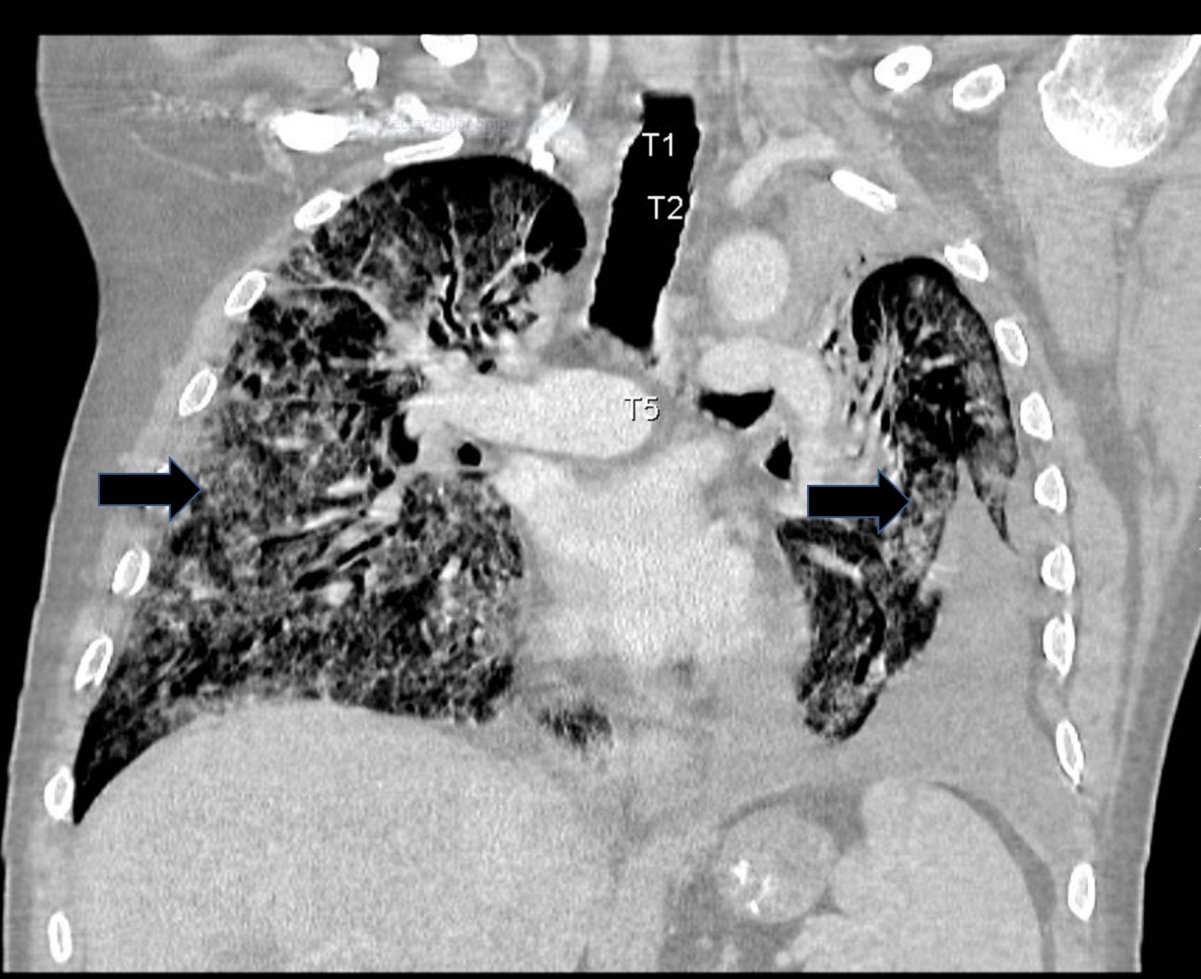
Computed Tomography of the Chest (Coronal View). Bilateral diffuse areas of ground-glass opacities involving the majority of the right and left lung consistent with interstitial pneumonitis.
